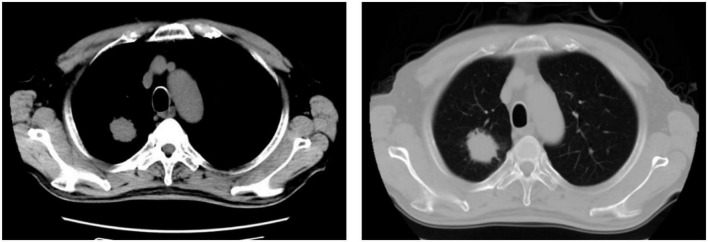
Chest computer tomography (CT) showed a space-occupying lesion in the right lung.
